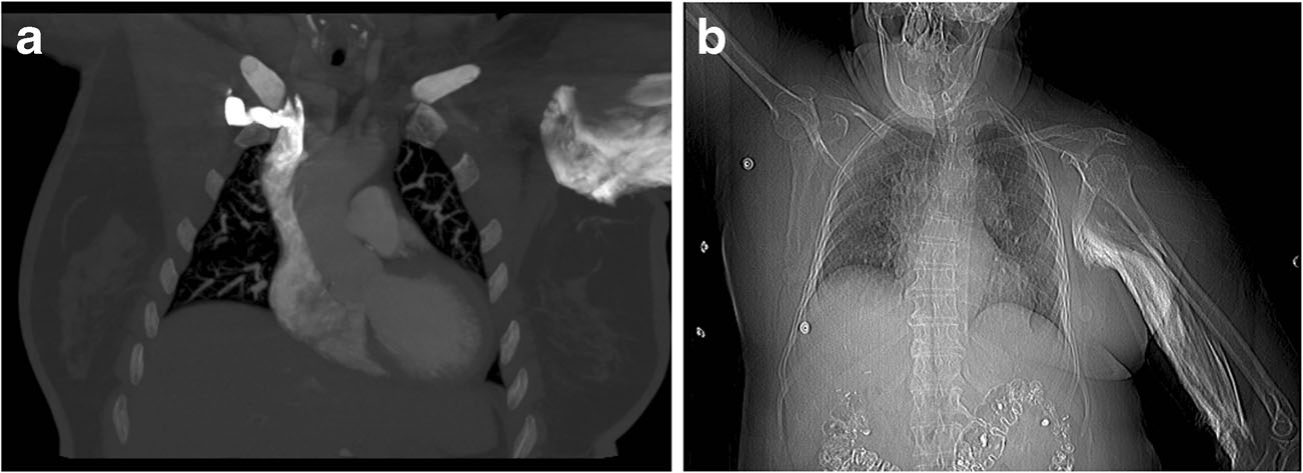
a Extravasation of intravenously injected contrast material. Coronal reformatted CECT images depicts extravasated contrast in the upper extremity soft tissues. b: CT scout image after extravasation of intravenously administered contrast in the left upper extremity allows assessment of the extent of extravasation and permits evaluation of possible compartment syndrome
